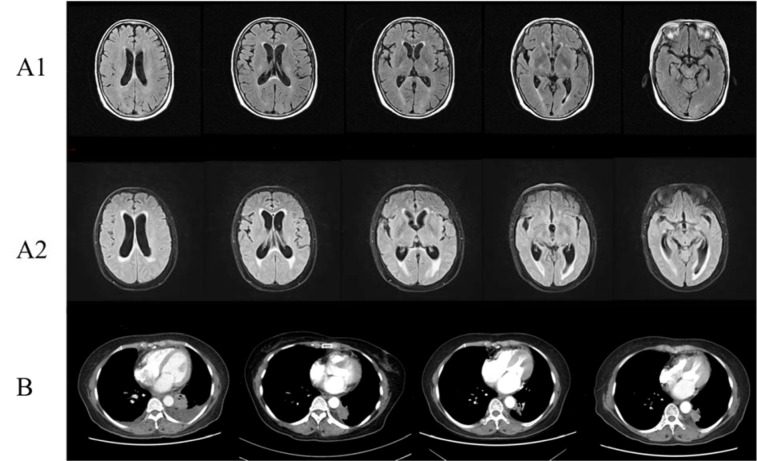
Clinical images of Case 4. (A1) Brain MRI showed some ischemic foci in the frontal lobe and slight demyelination before EGFR-TKI therapy; (A2) Brain MRI showed more ischemic foci in the frontal lobe, bilateral ventricle and basal ganglia region, lacunar infarction, demyelination, and encephalatrophy after EGFR-TKI therapy; (B) Chest CT with contrast of patient 4 showed partial response in chest lesions after EGFR-TKI treatment.
